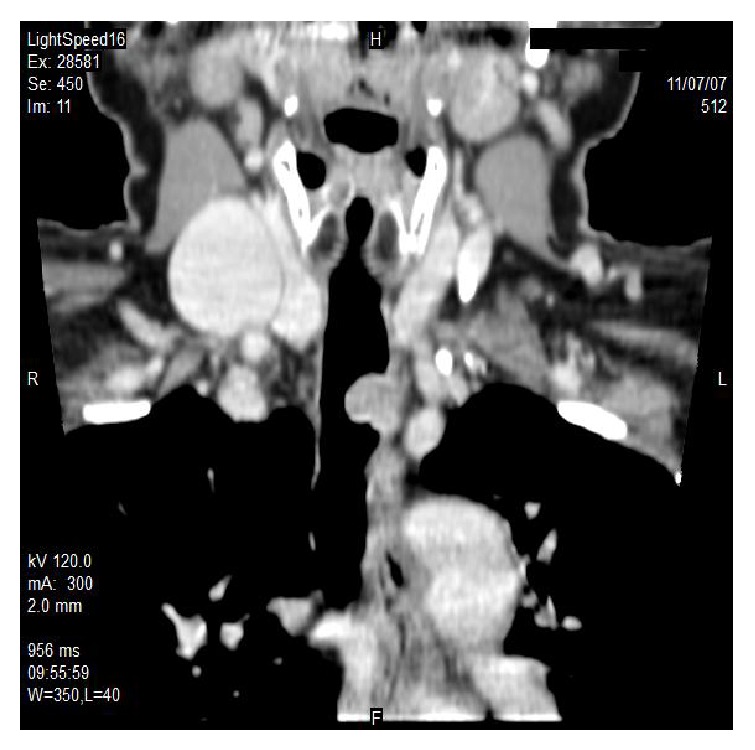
64-year-old female with granular cell tumor of trachea. Coronal contrast-enhanced CT scan (16-slice Multidetector CT, GE Medical Systems, venous phase) obtained at the level of the aortic arch shows an eccentrical mass (white arrow) growing in the left side of trachea, occupying half of the tracheal lumen.
